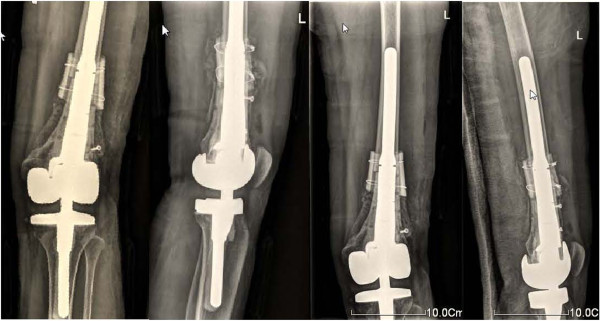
Postoperative radiographs showed good placement of the femoral and tibial prostheses.
